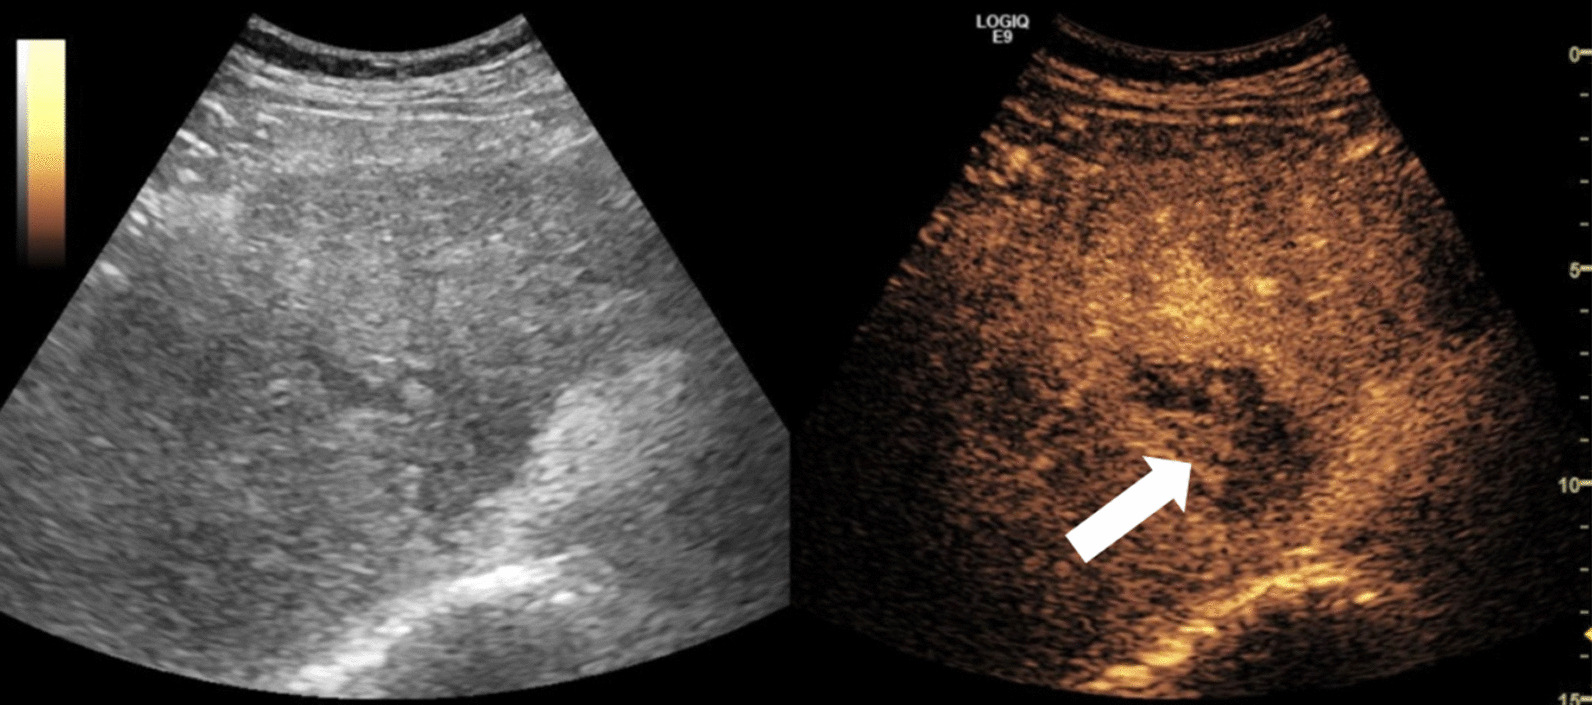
Example CEUS image from a male HCV patient with 4 cm HCC in segment 8 two weeks post transarterial chemoembolization. Nodular peripheral enhancement is observed on CEUS (white arrow), indicating residual viable tumor. Clinical contrast enhanced MRI was obtained 5 weeks post treatment and read as equivocal. Viable tumor was later confirmed via angiography during retreatment
